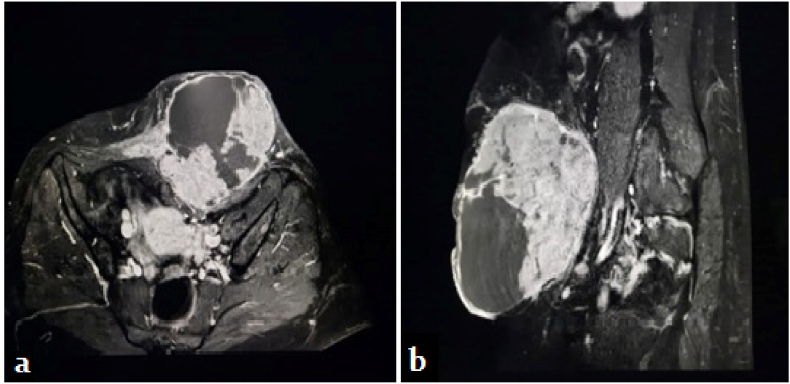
Abdominal MRI showing a subcutaneous mass developing at the expense of the anterior abdominal wall. (a) axial view. (b) sagittal view.
